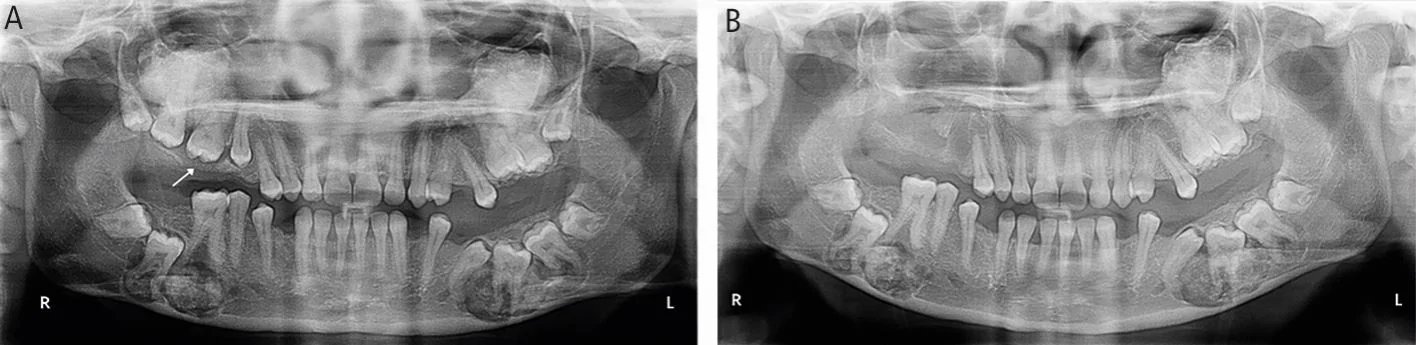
Panoramic radiographs of Case 1 (at 20 years old). (A) Focal bony discontinuity in the region of the crown of tooth 16 (arrow). (B) Radiographic appearance 22 days after surgical removal of the lesion in the right maxilla and extraction of 15, 16, 17, and 18
